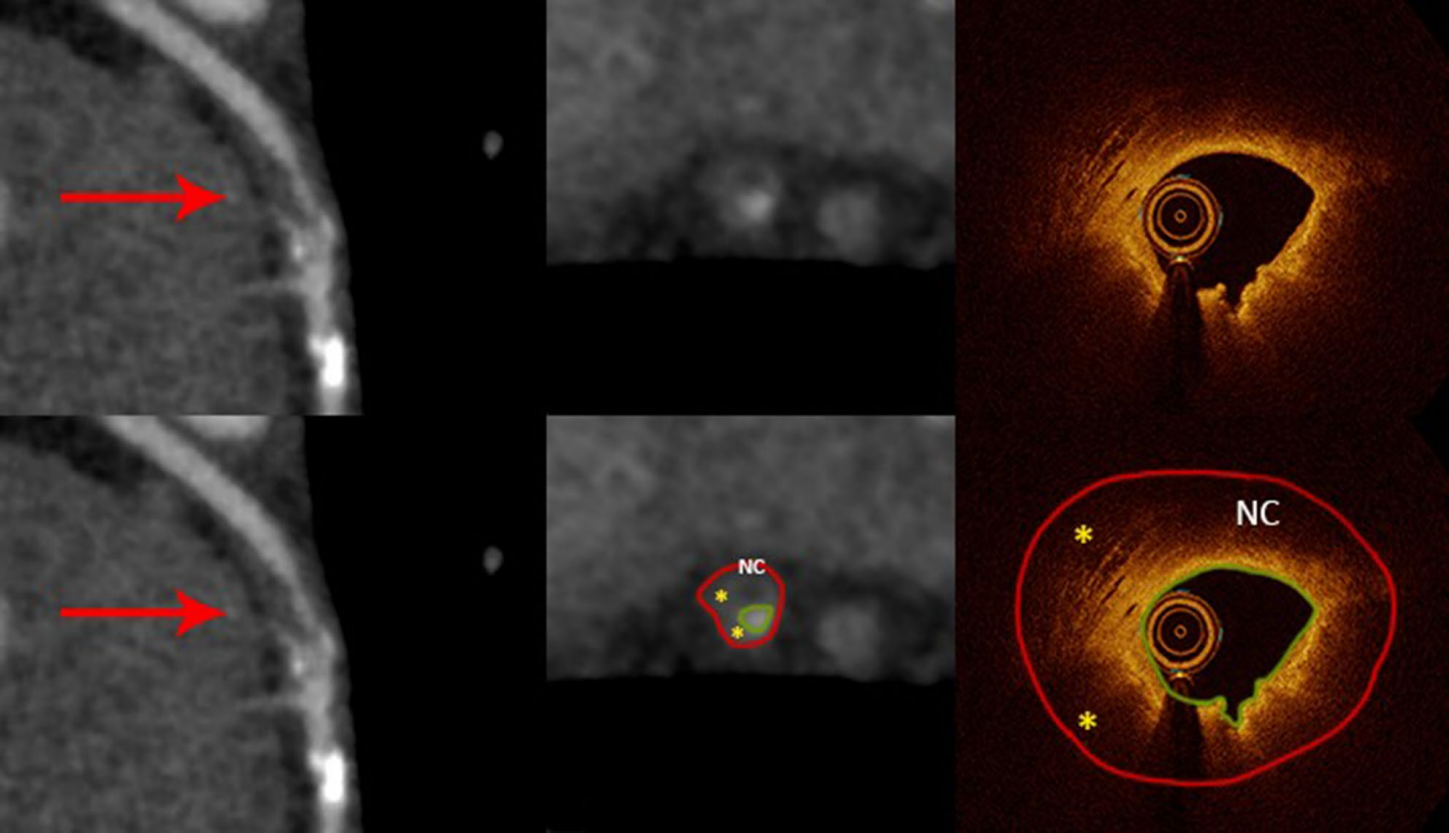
Curved multiplanar reformation computed tomographic images (left) and cross-section (middle) views of the left anterior descending artery from CCTA (red arrows) demonstrates hypoattenuated lesions with a dark core (yellow asterisks) giving rise to a ‘napkin ring’ appearance. The corresponding OCT image (right) reveals luminal irregularity with fibrofatty plaque (yellow asterisks) and a necrotic core (NC), which appears to match the dark core on CCTA (lower middle). The red contours encompass the vessel wall and the green ones outline the vessel lumen.
